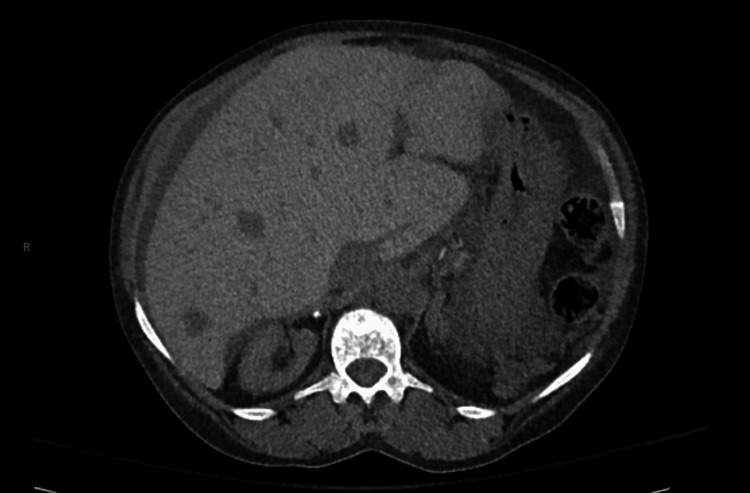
Abdominal CT, axial view, demonstrating hepatomegaly
